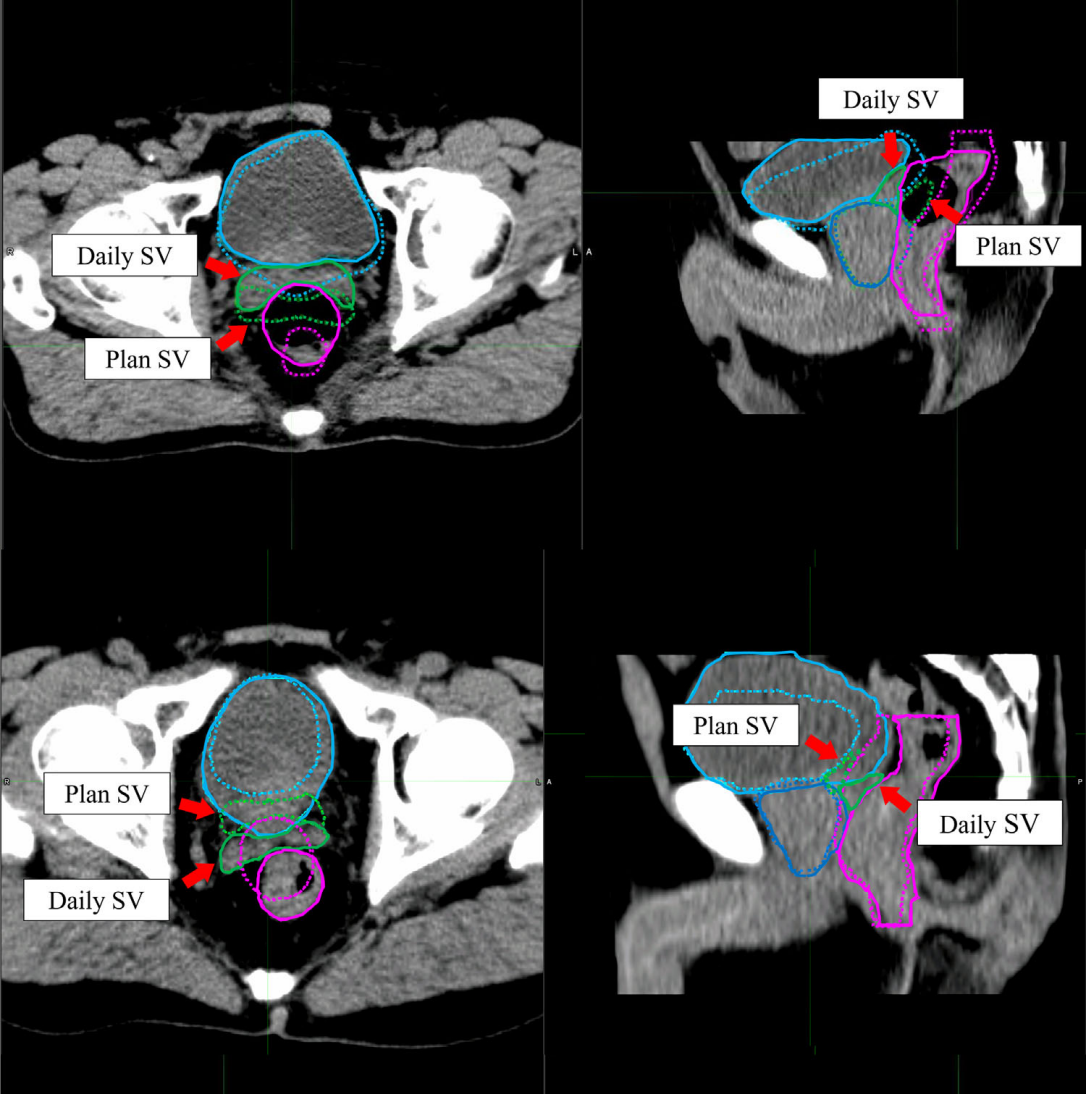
Inter‐fractional seminal vesicle (SV) positional deviations due to the rectal and bladder volume variations are shown. The upper and lower panels show the volume variations of rectum and bladder, respectively. The daily regions of interest (ROI) (solid line) and the original plan ROI (dashed line) are shown on the daily computed tomography (CT) image in the prostate (blue), seminal vesicle (SV) (light green), rectum (magenta), and bladder (light blue)
